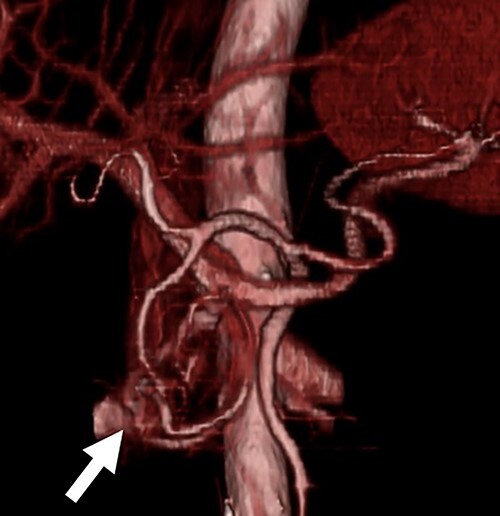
Three-dimensional CT image. The three-dimensional CT imaging showed the communication between arteries and veins in the pancreatic head (arrow).
